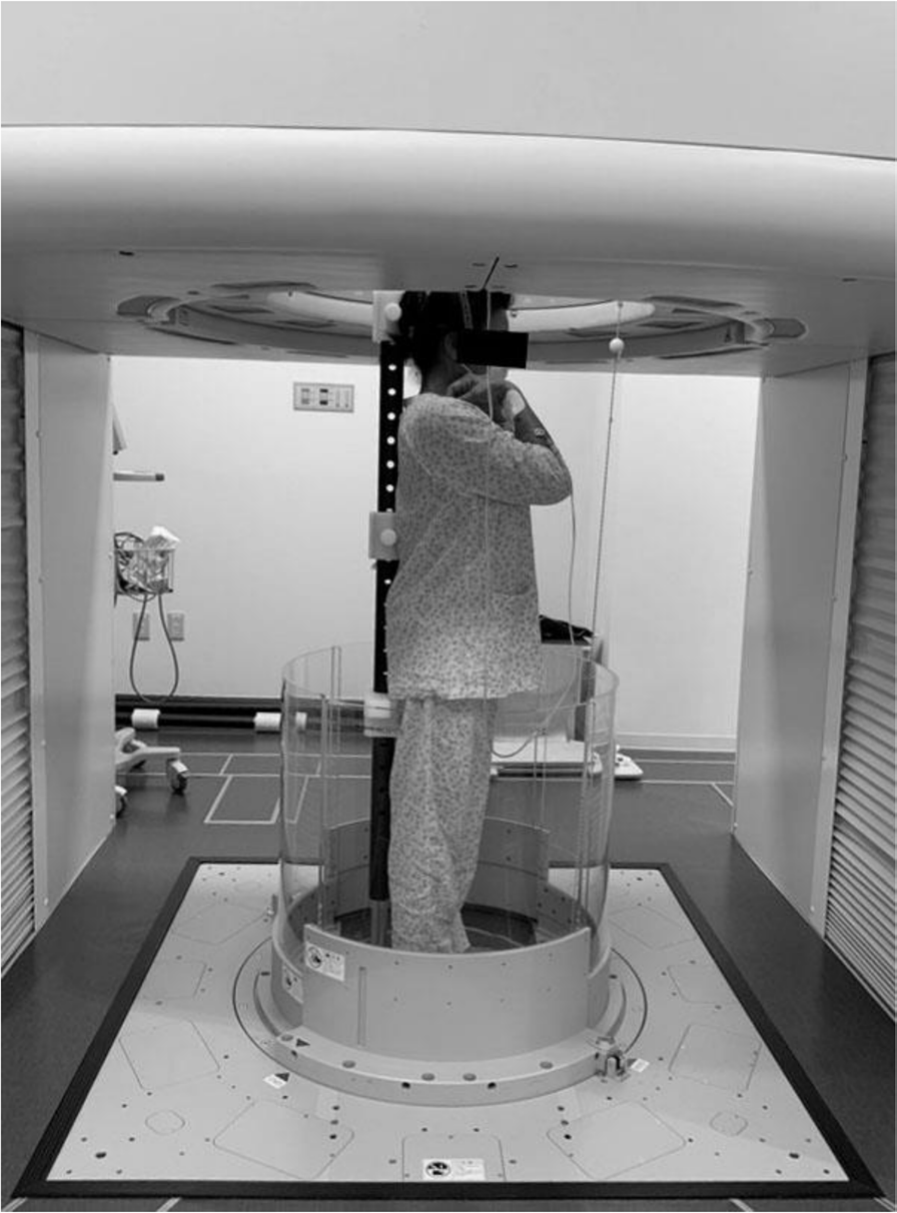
The 320-row upright CT scanner . (Prototype TSX-401R; Canon Medical Systems, Otawara, Japan). During CT scan, the patient’s back was partly touched by the pole for patient safety
